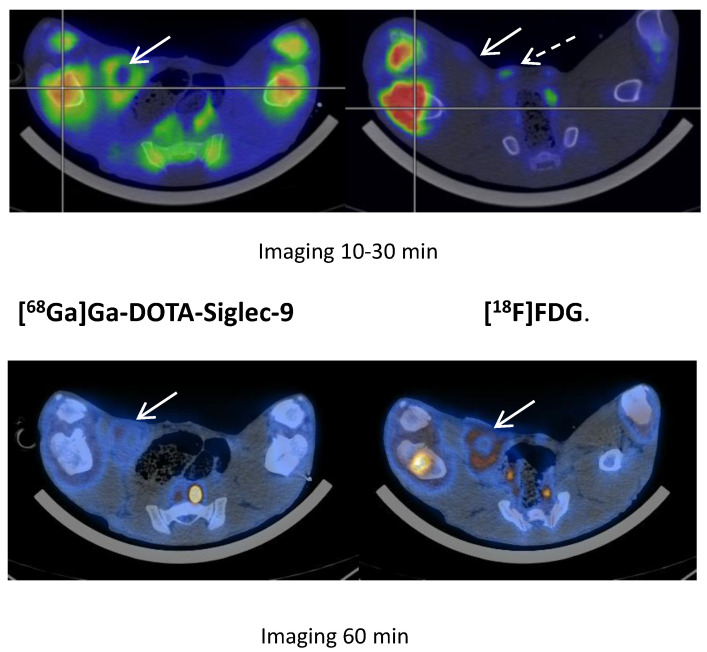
[^68^Ga]Ga-DOTA-Siglec-9 compared to [^18^F]FDG.
